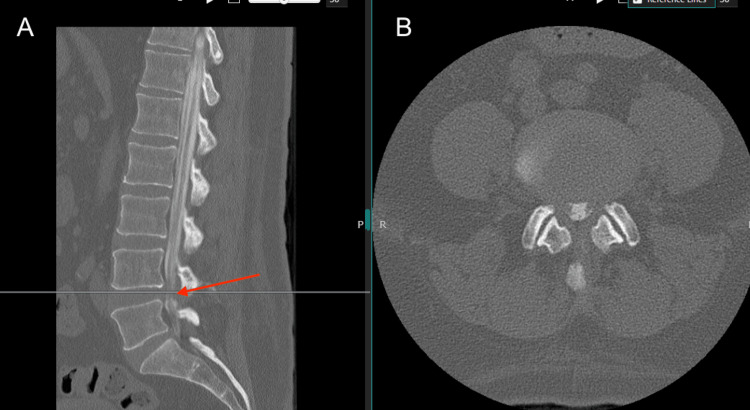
Preoperative CT Myelography Preoperative sagittal (A) and axial (B) myelography showing lumbar spinal stenosis at the level of L4/L5
